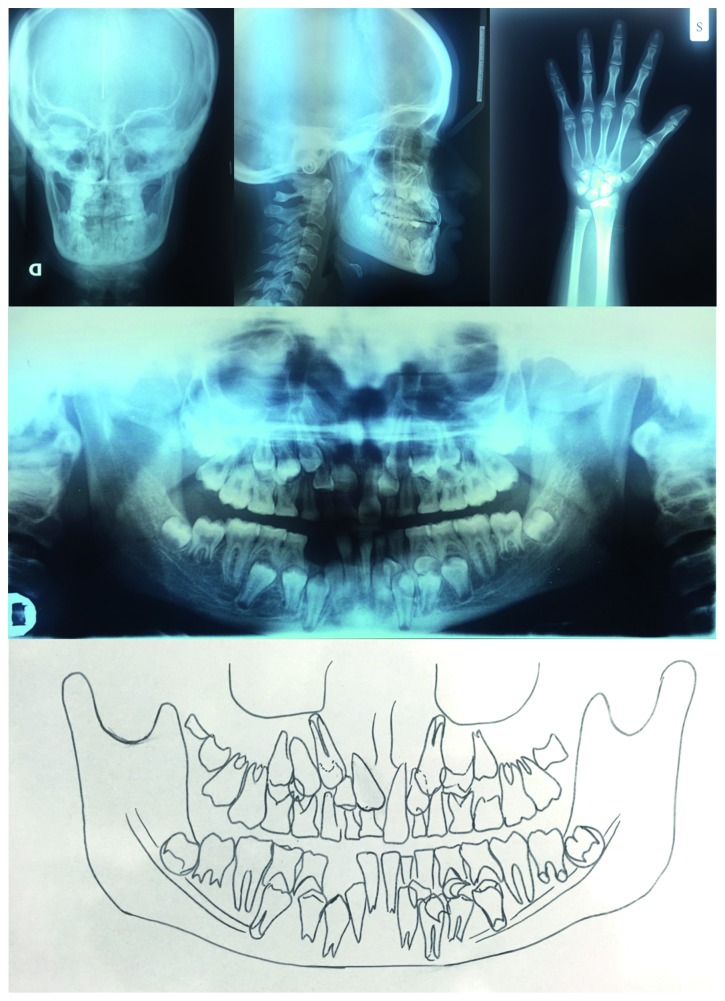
Radiographs of skull and panorex.
